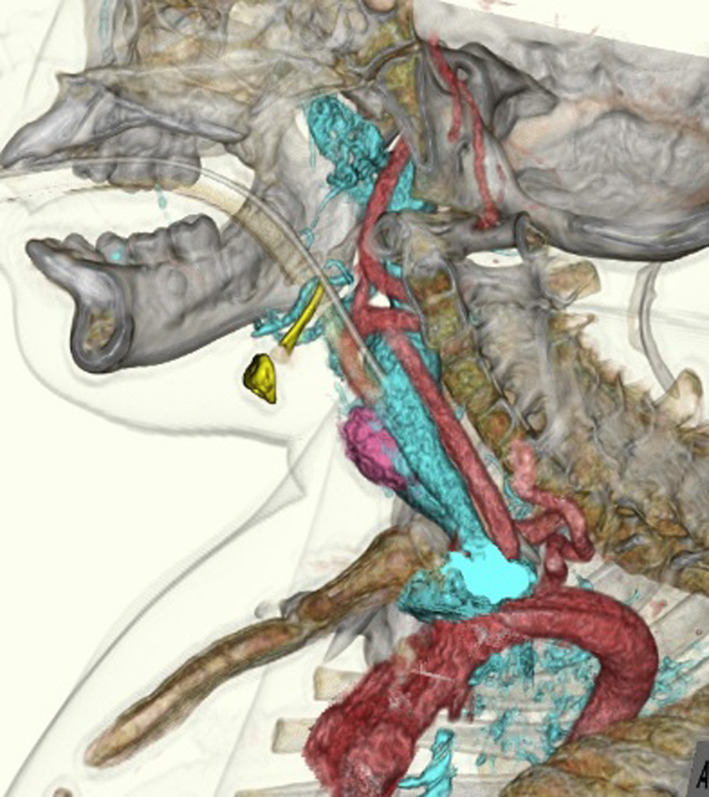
Three-dimensional computed tomography (3D-CT) before tracheostomy
